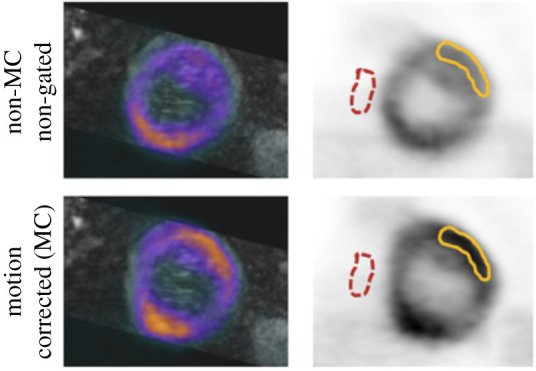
Identifying regions affected by motion: blurring and signal loss within the myocardium when non-motion corrected (non-MC) non-gated compared to motion corrected (MC) PET images as in the antero-lateral wall (solid line). Background regions were drawn in the blood pool on the right or left ventricle (dashed line). (Reproduced from Robson PM, Trivieri MG, Karakatsanis NA, Padilla M, Abgral R, Dweck MR, Kovacic JC, Fayad ZA. (2018) Correction of respiratory and cardiac motion in cardiac PET/MRI using MR-based motion modelling. *Physics in Medicine and Biology*
